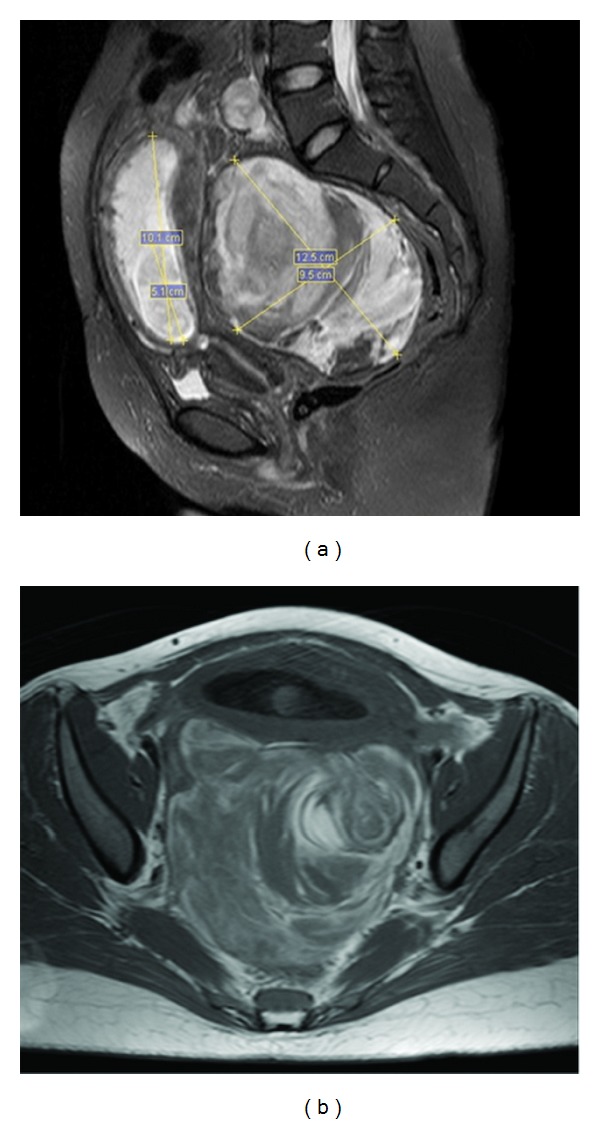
(a) T2-weighted sagittal sequence MRI, showing uterus with 12-week conception in the anterior region and a complex mass occupying the retrouterine region; (b) adnexal T2-weighted sequence MRI, showing large cystic mass with “onionskin” appearance, which might correspond to multiple episodes of organized bleeding. MRI: magnetic resonance imaging.
